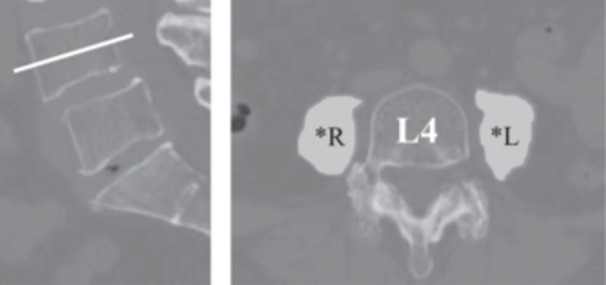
Cross‐sectional area of the right (*R) and left psoas major (*L) at the mid‐level of the L4 vertebral body, determined by manual tracing of preoperative computed tomography images. The sum of these areas represents the total psoas muscle area, which correlates positively with total skeletal muscle volume, serving as a prognostic indicator for sarcopenia.
